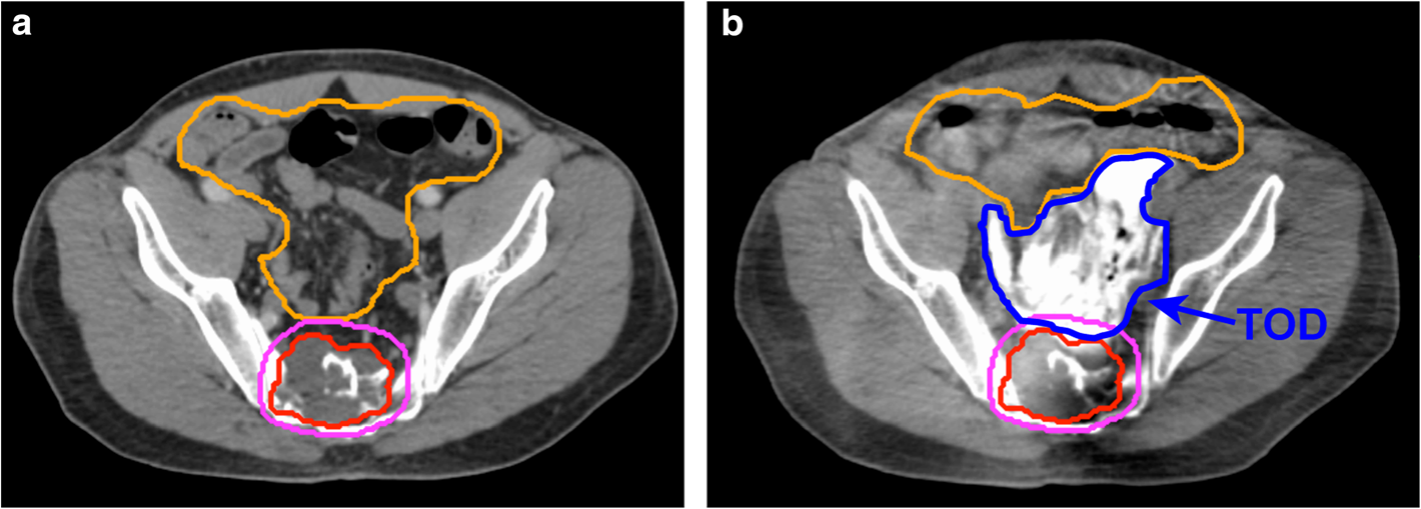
Axial imaging of a sacral chordoma treated to 2400c Gy. (a) Baseline computed tomography (CT) scan revealing pelvic sacral tumor target adjacent to bowel at S2-4. (b) Cone-beam CT confirming temporary organ displacement (TOD) bowel. TOD is well visualized as a hyperdense region (dark blue) between the planning target volume (pink) and bowel (orange). CBCT enables calculation of dose delivered to the critical structures.
